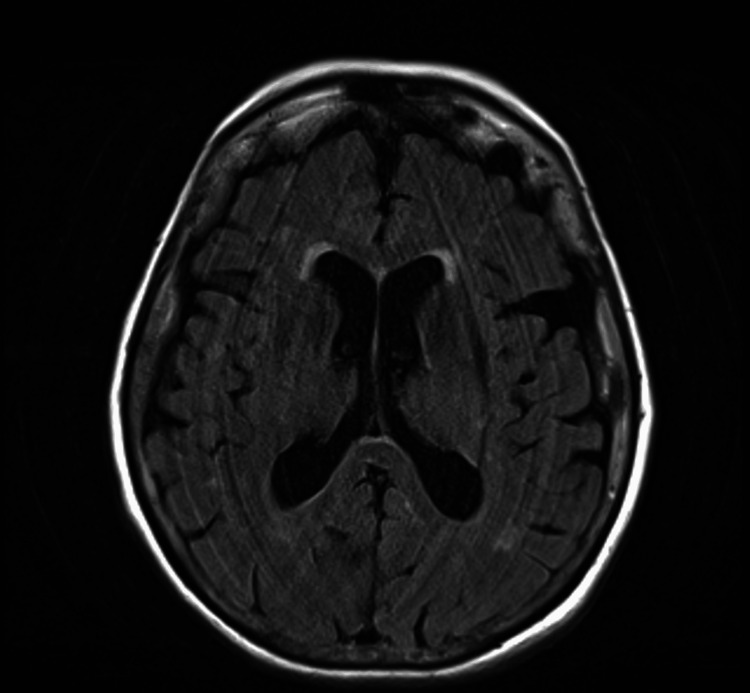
MRI brain showing absence of acute stroke MRI: magnetic resonance imaging
